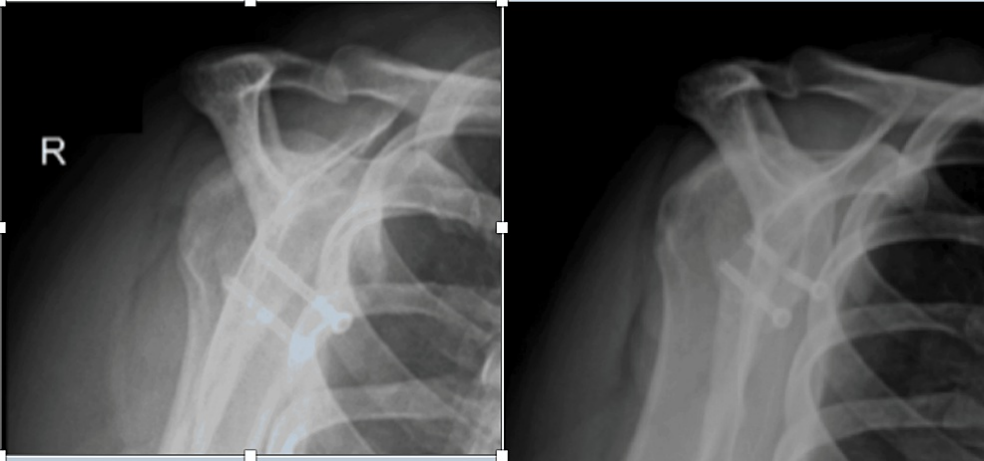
Scapular Y radiographic view at 1 week (left) and 4.5 months (right) after the distal tibial allograft procedure.
